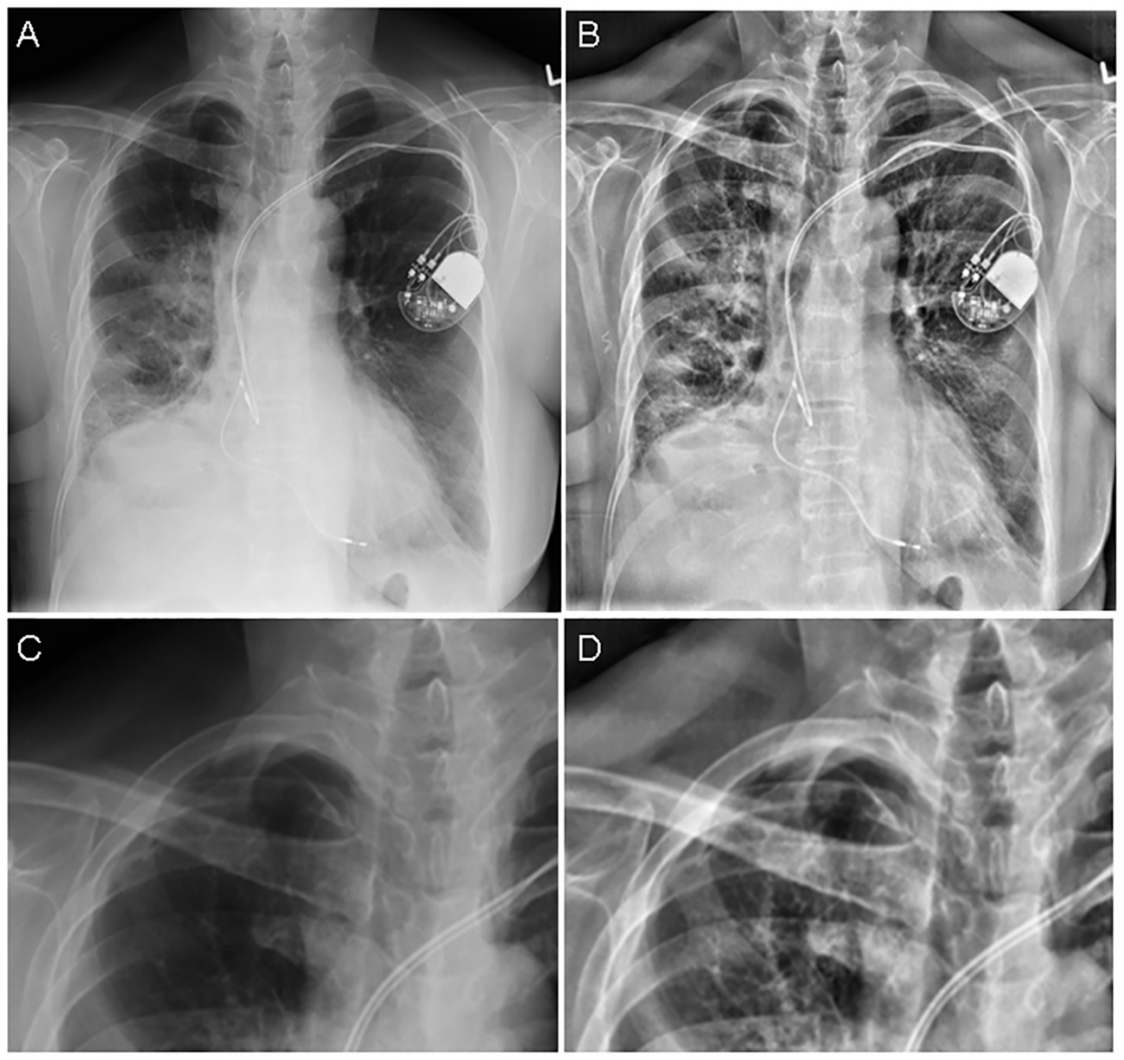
Portable chest radiograph of intensive care unit patient demonstrating the appearances of the conventional (A) and edge enhanced (B) images with corresponding magnified views demonstrating a small right apical pneumothorax (C, D).
